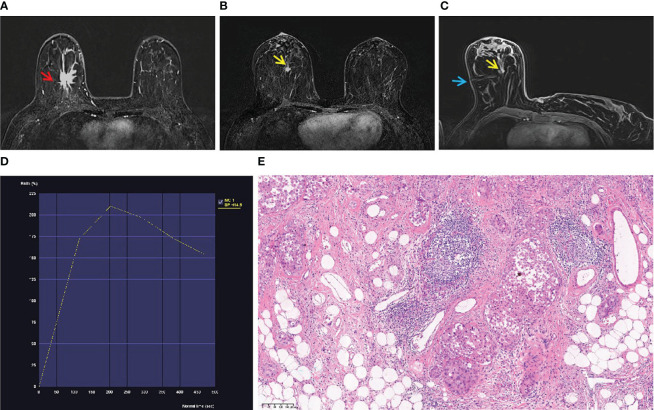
A 53-year-old woman with palpable mass in the right breast. (A) An irregular mass (red arrow) located in the right breast (BI-RADS 5) was shown in T1-weighted fat-suppressed contrast-enhanced MRI. It was confirmed as invasive breast cancer by puncture pathology. (B) There was a focal lesion (yellow arrow) below the mass in the right breast, and the BI-RADS category was considered as 4B. It was not found by ultrasound or mammography. (C) The lesion (yellow arrow) was performed by MR-guided wire localization (blue arrow). (D) The dynamic contrast-enhanced time–signal intensity curve (TIC) appeared as “wash-out type”. (E) The lesion was confirmed as low-grade intraductal carcinoma.
